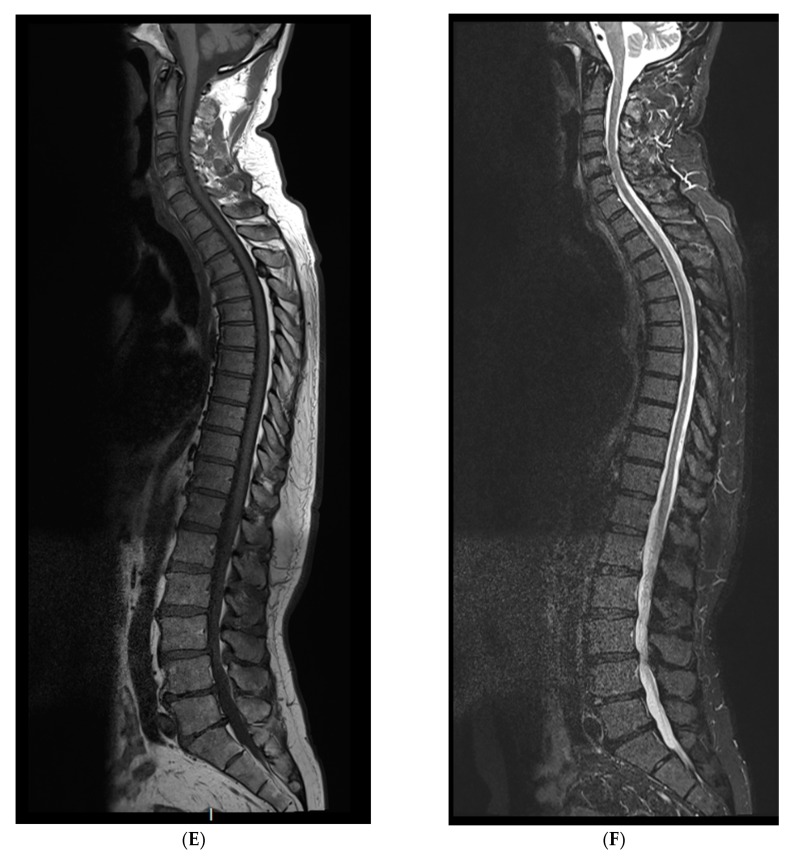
IgGk smoldering multiple myeloma (SMM) patient with negative whole-body computed tomography. Magnetic resonance imaging of the rachis and pelvis shows two focal bone lesions with increased signal intensity in T2-weighted STIR (short-tau inversion recovery) images. One small lesion (diameter: 5 mm) of the right iliac crest (A) and one bigger lesion (diameter: 14 mm) of the right acetabulum anterior column (B), with corresponding enhancement after Gadolinium injection in T1-weighted images with fat saturation (C,D). No further focal lesion of the rachis was detected in whole-rachis sagittal T1-weighted and T2-weighted STIR sequences (E,F). These two focal bone lesions are considered a myeloma-defining event leading to the recommendation to treat this patient.
